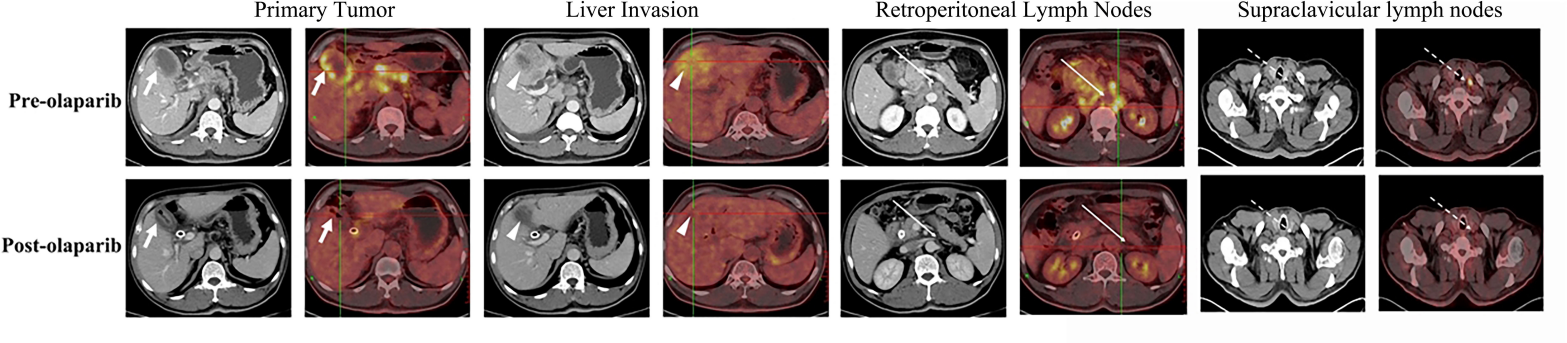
Representative pre- (upper row) and post-olaparib (lower row) treatment showing radiologic evidence in a 52-year-old man with gallbladder cancer harboring BRCA1 mutation. After 2 months of olaparib treatment, extensive metastatic lesions that involved the primary tumor (white arrow) and liver invasion (white arrowheads) became markedly reduced, while the retroperitoneal lymph nodes (long white arrows) and left supraclavicular lymph nodes (long white dotted arrows) disappeared when compared with those in the pre-olaparib treatment CT and PET/CT images.
